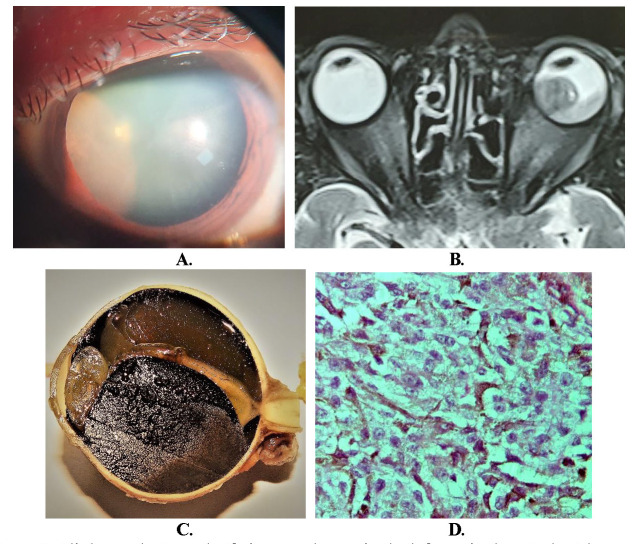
A. Slit lamp photograph of pigmented mass in the left eye in the retrolental area on dilatation of pupil; B. T2-weighted Magnetic Resonance Imaging (MRI) of brain and orbit in axial view showing a mushroom shaped hypointense large malignant choroidal melanoma;
